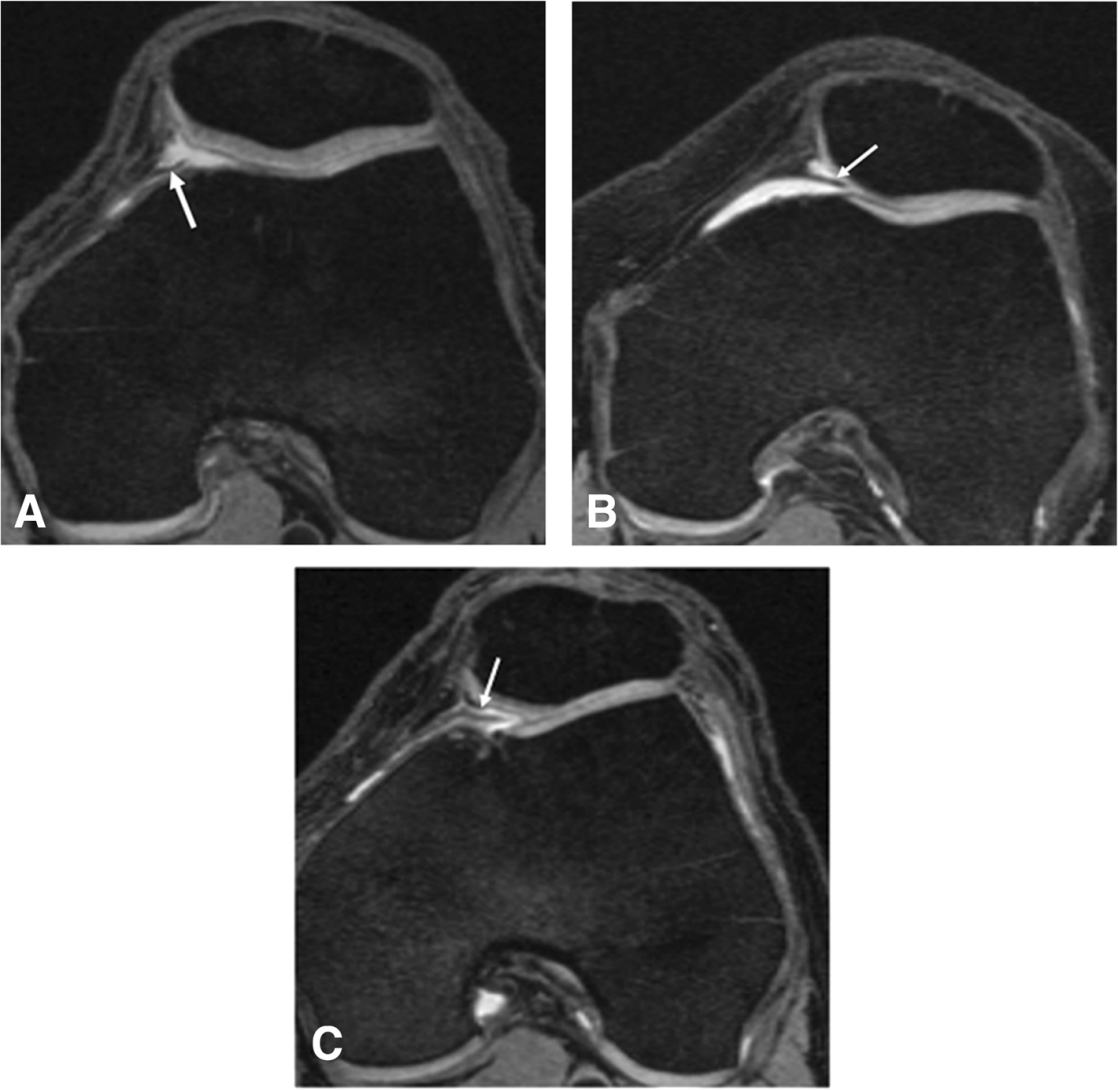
MRI classification scheme of mediopatellar plicae modified from the Sakakibara arthroscopic classification. (A) Type A lesion in the left knee consisting of a cord-like elevation in the synovial wall; (B) Type B lesion in the left knee, which has a shelf-like appearance but does not cover the anterior surface of the medial trochlea; (C) Type C lesion in the left knee, which has a large shelf-like appearance and covers the anterior surface of the medial trochlea.
